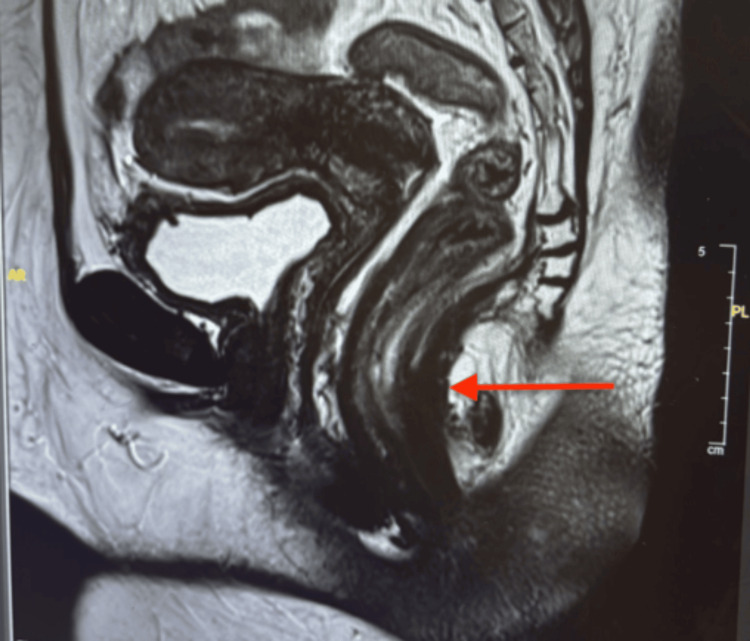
Pre-surgical MRI of the pelvis shows that the perianal region, anus, and rectum appear normal, with no evidence of wall thickening or masses (arrow).
